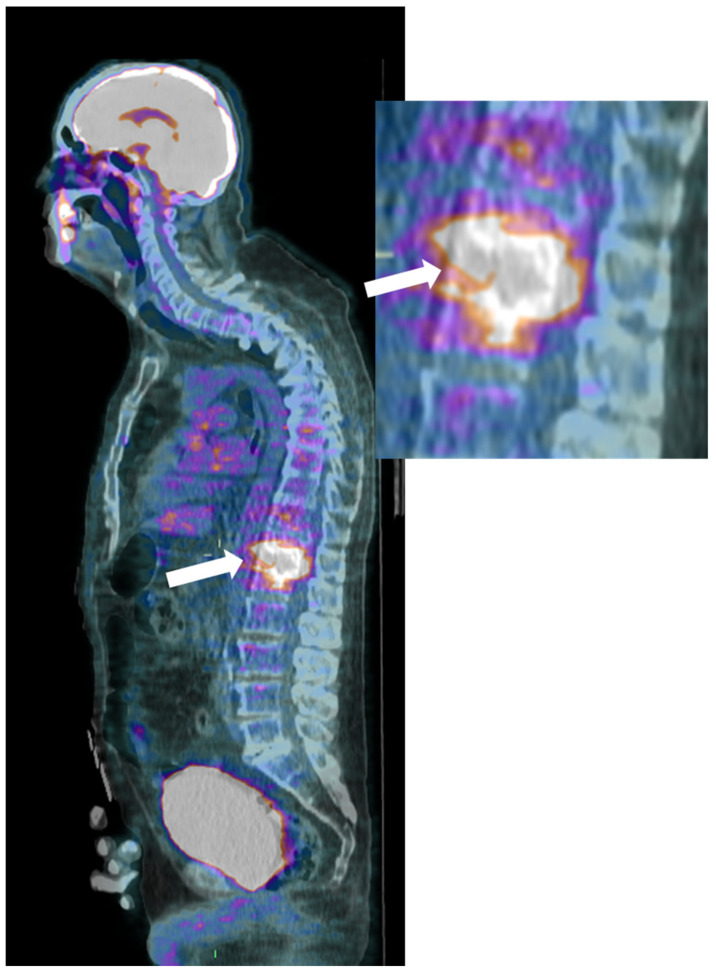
(FDG) PET-CT, Sagittal reconstruction (magnification on the right) of a 72-year-old male with pyogenic spondylodiscitis of T12-L1 vertebrae. A high pathologic FDG uptake is detected in the disc and vertebral endplates (arrows).
